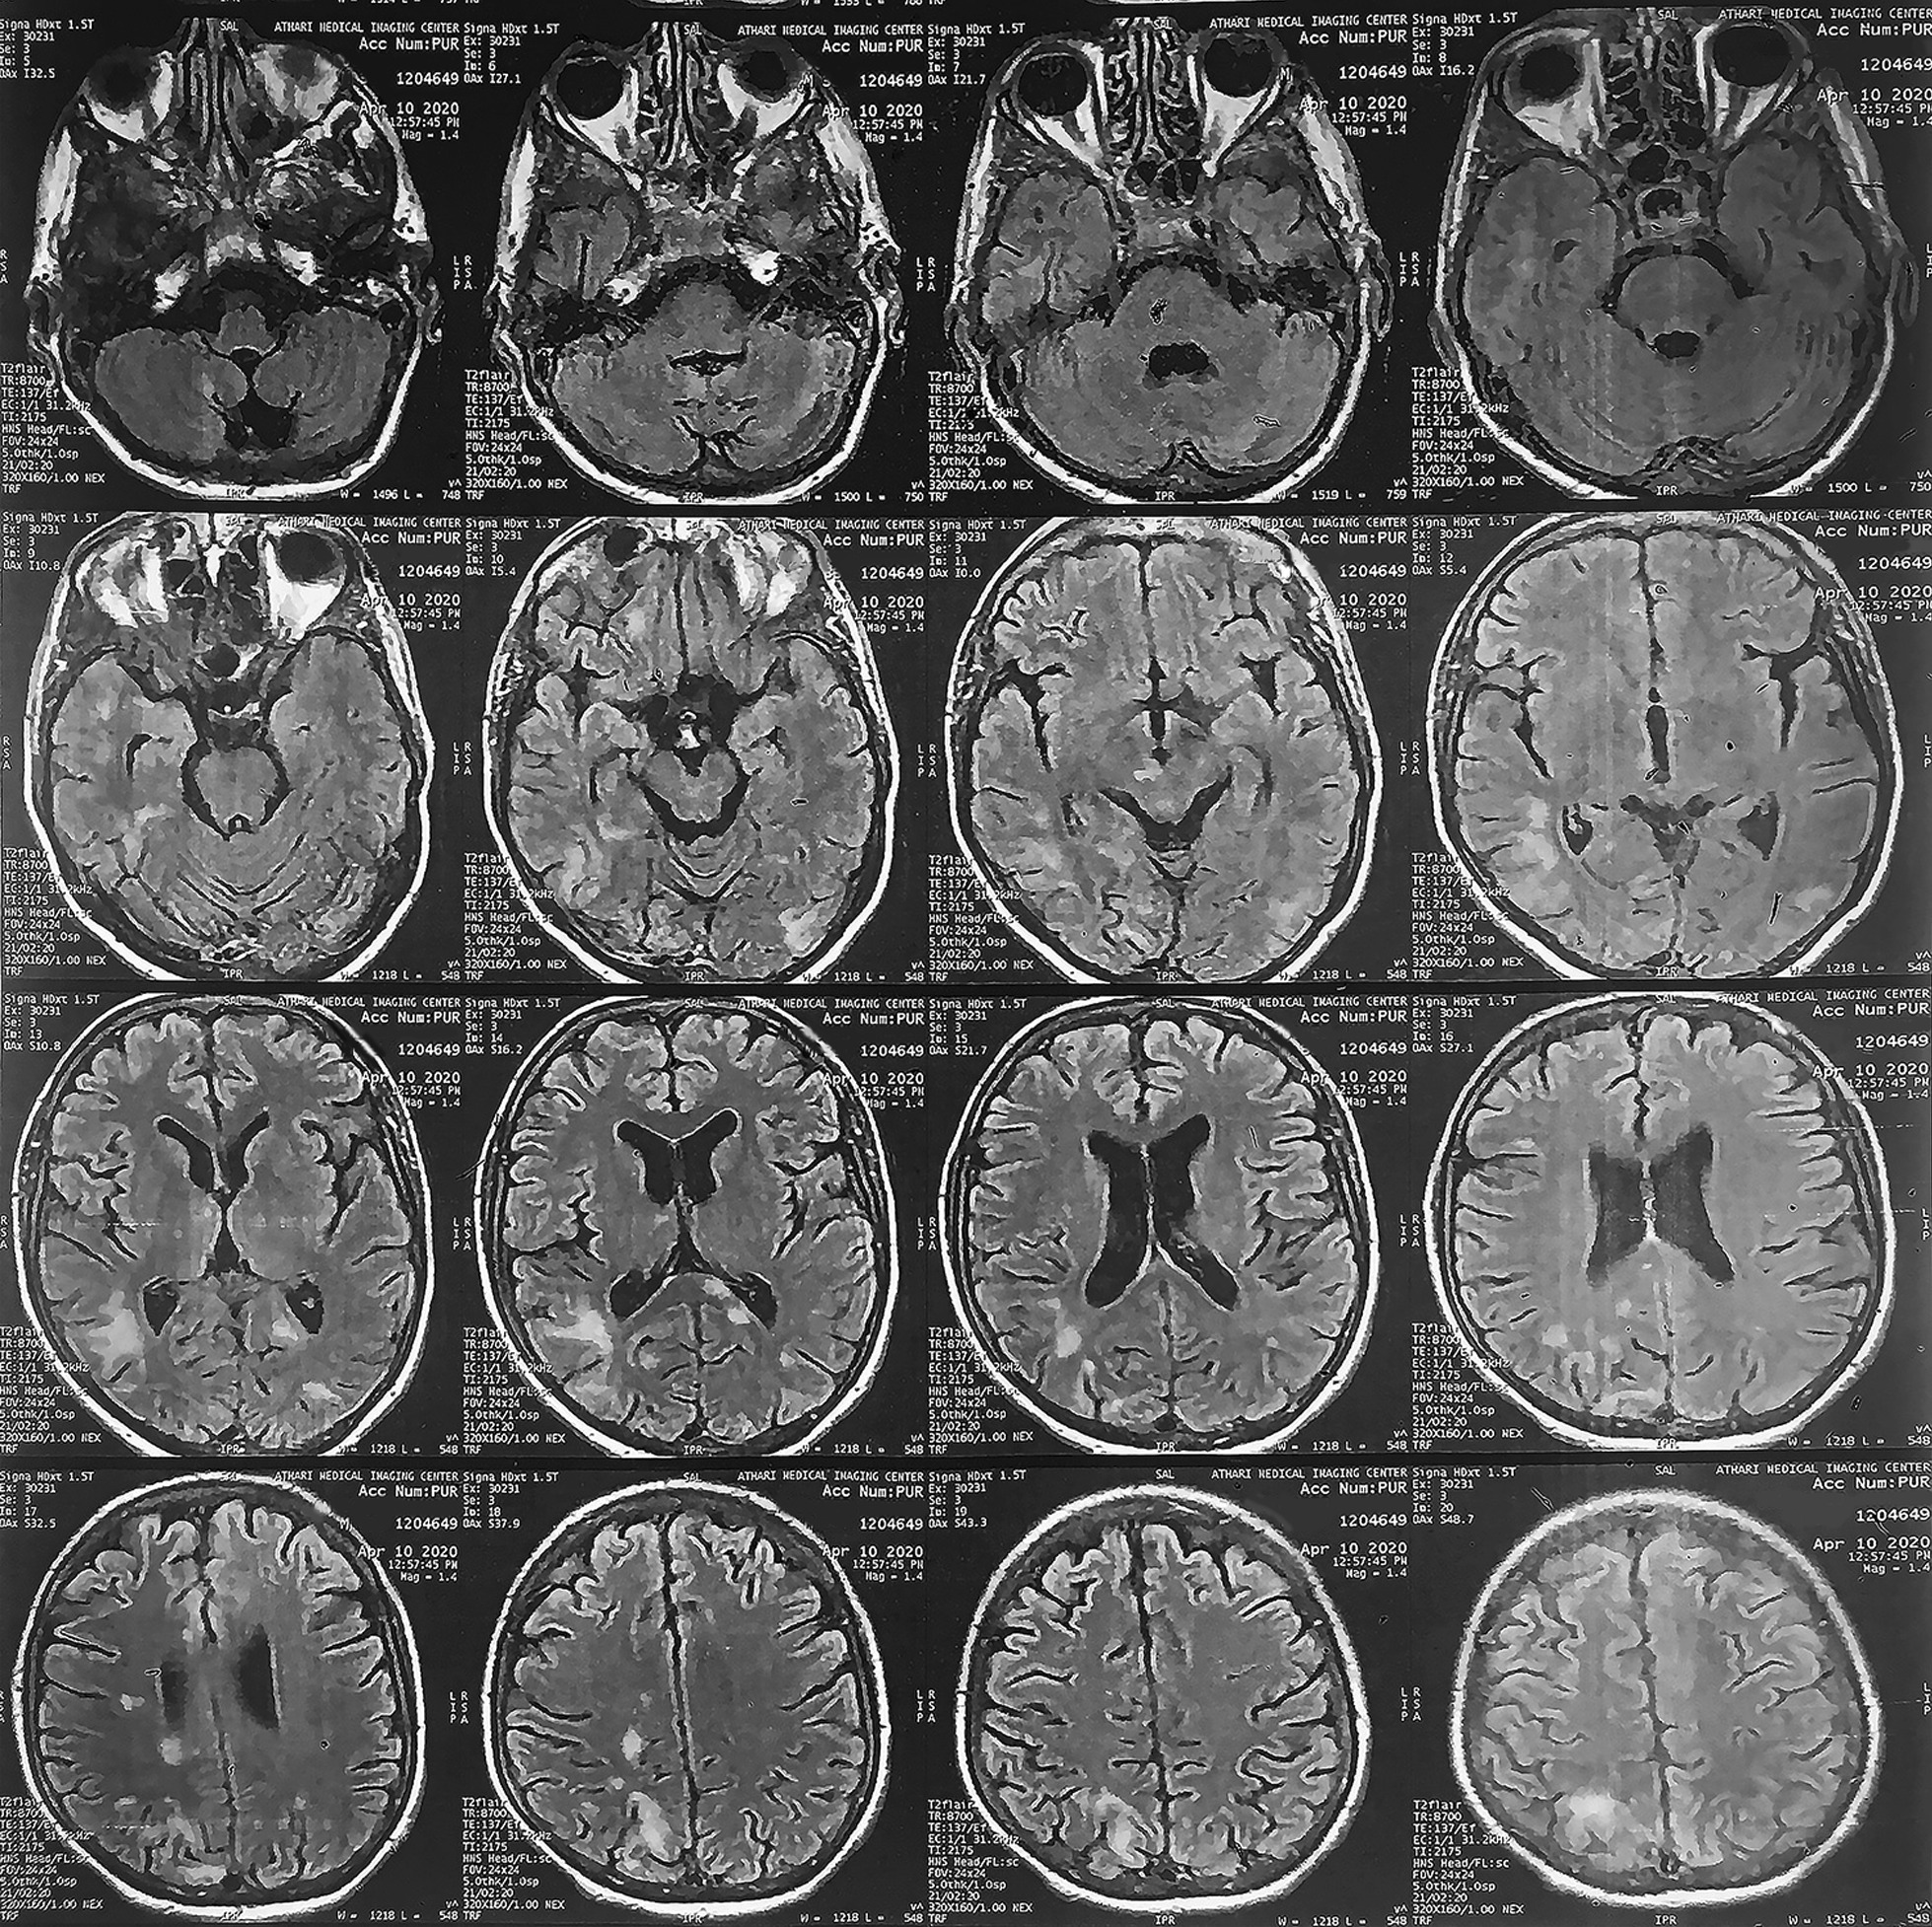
Magnetic resonance imaging of the brain, showing subcortical signal changes in the left occipital and right temporoparietal area
